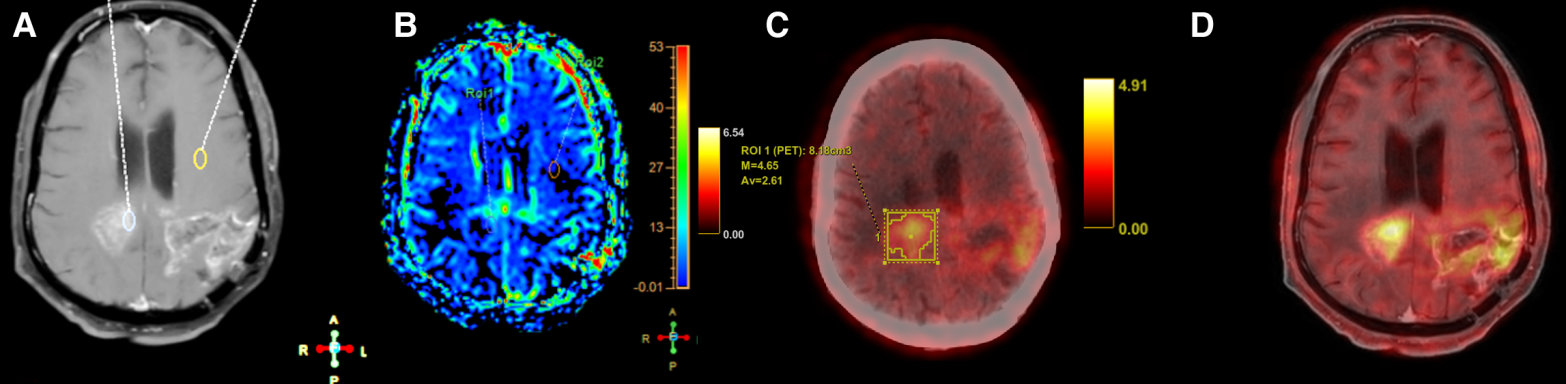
Axial T1 + Gd (A), perfusion map (B), FET-PET (C) and fused MRI and FET-PET images in a case of glioblastoma shows areas of thick nodular enhancement in bilateral posterior parietal lobes with hyperperfusion (B). FET-PET (C) shows significant uptake (T/W ratio: 3.6). Fused images (D) shows corresponding areas of increased perfusion and uptake, suggestive of recurrence.
